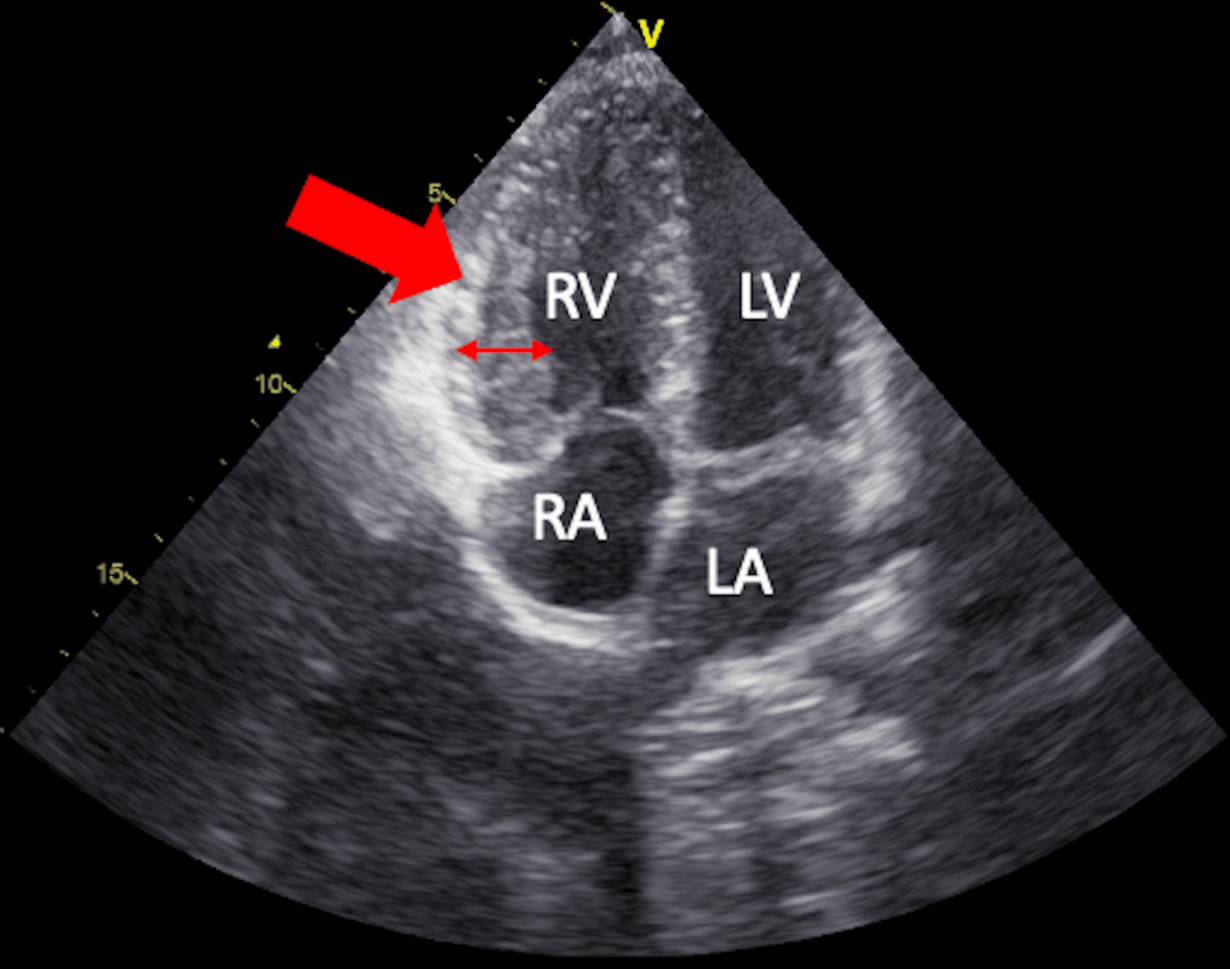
Apical Four-Chamber TTE Showing Right Ventricular Hypertrophy Right ventricular hypertrophy (red arrow) is seen with preserved cavity size. The left-sided cardiac chambers appear normal. These findings reflect chronic right ventricular pressure overload secondary to severe pulmonary valve stenosis. RV: right ventricle; LV: left ventricle; RA: right atrium; LA: left atrium; TTE: transthoracic echocardiography
